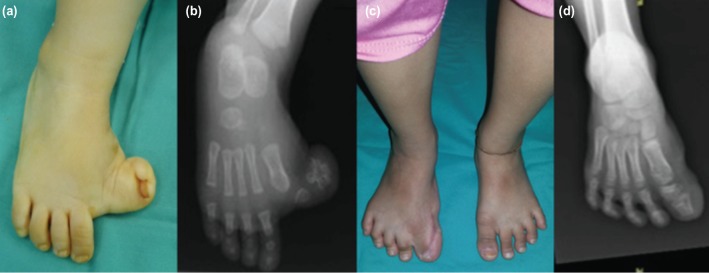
(a) Pre-operative photograph of case No. 1 shows a severe right hallux varus with wide first web space and polysyndactyly of the right big toe. (b) Radiograph shows a bifid distal phalanx of the right hallux. (c) Post-operative photograph after Farmer’s procedure with creation of syndactyly of the first web space and realignment of the right big toe. (d) Post-operative radiograph shows a well reduced first metatarsophalangeal joint and a corrected right hallux varus.
